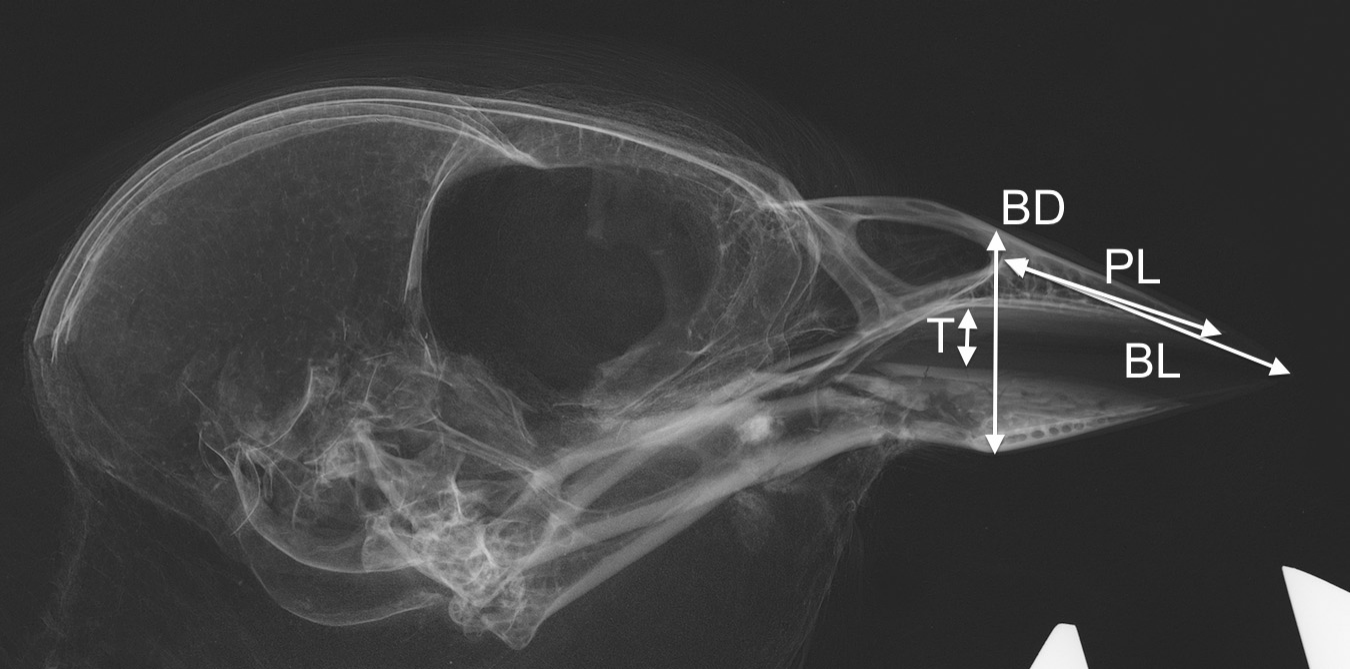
Radiograph of a sparrow showing the measurements taken for calculations of skeletal bill size: BL = Bill Length; PL = Premaxillary Length; BD = Bill Depth; T = Tomium.
